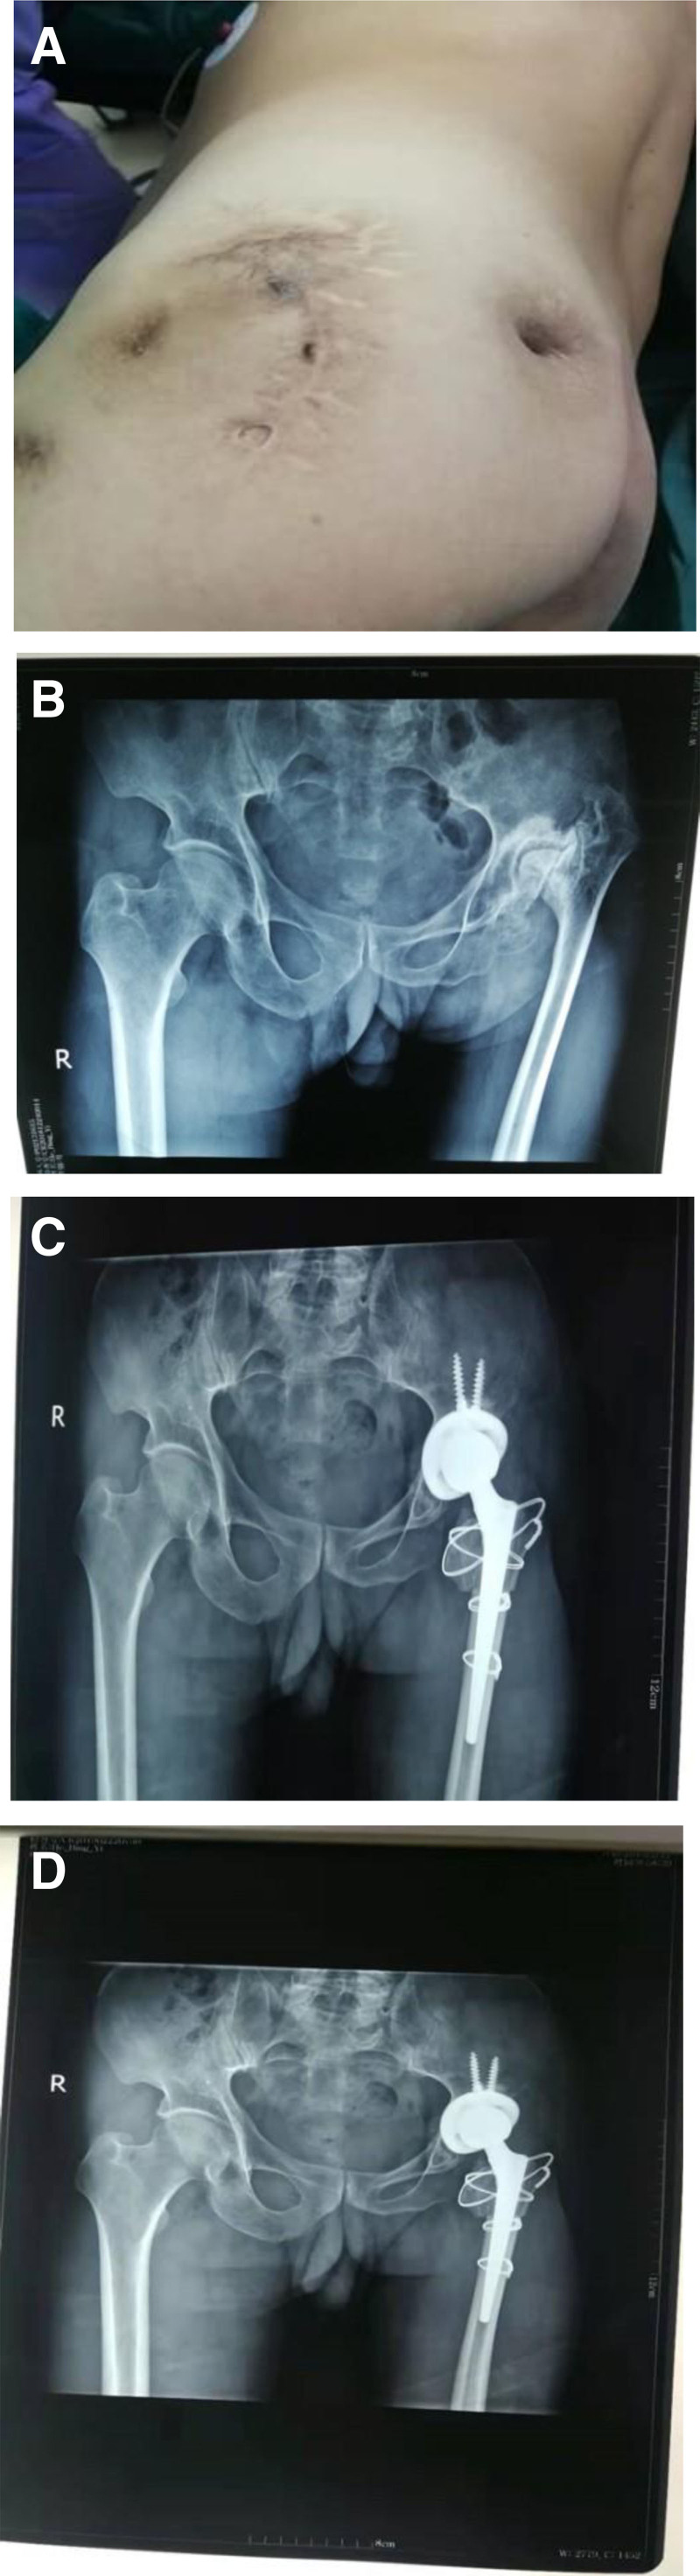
(A) Preoperative photos showed multiple wounds and sinus scars. (B) Preoperative radiographs showing missing left femoral head. (C) Postoperative radiographs, subtrochanteric osteotomy. (D) Radiographs 1 year after surgery showed that the osteotomy had healed.
